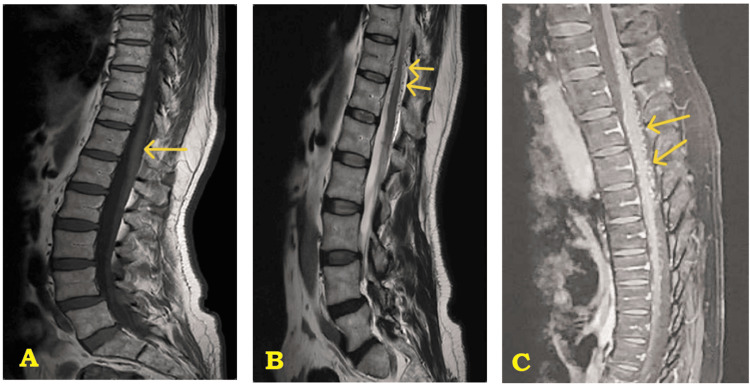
Sagittal thoracolumbar spine MRI demonstrating diffuse long-segment spinal cord signal abnormality (edema of the medullary cone and flow voids). (A) T1-weighted sagittal sequence: intramedullary hypointense signal; medullary cone enlargement: edema (arrow). (B) T2-weighted sagittal sequence: hyperintense signal in the medullary center on T2, with hypointense tortuous structures in the perimedullary vein (arrows: tortuous flow voids). (C) T1 with gadolinium injection-weighted sagittal sequence: hyperintense tortuous structures in the perimedullary vein (arrows: tortuous flow voids).
